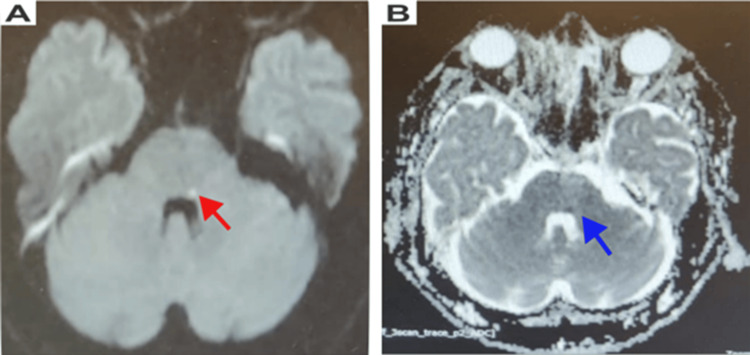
(A) MRI shows increased focus on DWI and (B) ADC restriction sequences in the dorsal aspect of the left pons at the floor of the fourth ventricle, corresponding to the left facial colliculus The small hyperintense spot in the DWI image is indicated by a red arrow, and the hypointense signal in the ADC image is shown by a blue arrow MRI: magnetic resonance imaging; DWI: diffusion-weighted imaging; ADC: apparent diffusion coefficient
